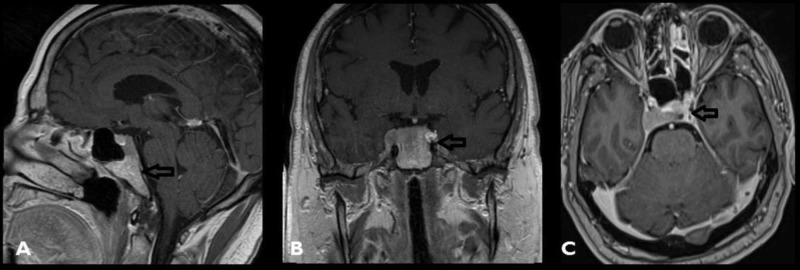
Different sections of an MRI T1 with a homogenous enhancing lesion involving the clivus (A-C); tumor invading right cavernous sinus (B, C)
